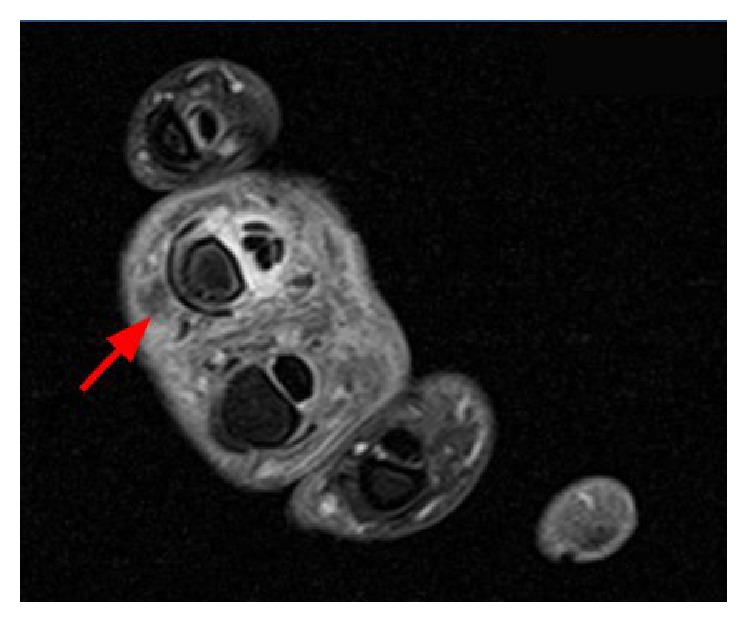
MRI axial view, postcontrast tenosynovitis.
